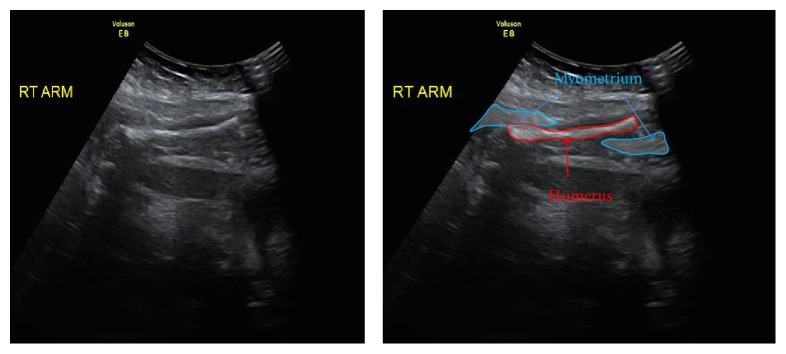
Fetal ultrasound showing uterine rupture and intra-abdominal right fetal arm.
